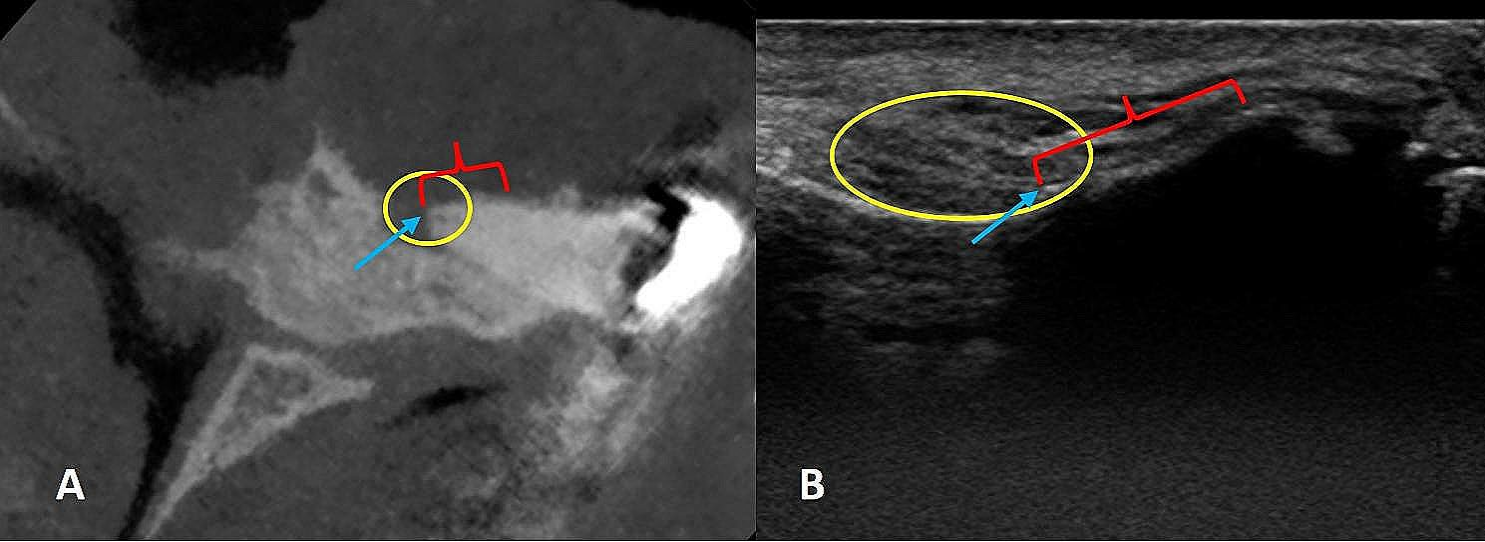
(A) CBCT image of a patient included in the study (which was taken for a different reason) shows a periapical lesion related with apex of right santral incisor teeth on cross-sectional view. (B) US image of the same tooth. The blue arrows indicate the root apex, the yellow circles denote the periapical region, and the red indicators define the root of the tooth. The CBCT image reveals a periapical lesion and bone resorption. In the US image of the same area, due to bone resorption, the ultrasonic wave has been transmitted the root and reflected back. Hence, the root apex (blue arrows) can be observed as hyperechoic within the apical lesion (yellow circle). Also, due to bone resorption in the relevant area, the ultrasonic wave has been able to reach the apical third of the root and reflected back. Therefore, the line indicated by the red marker appears more hyperechoic, while behind the opaque line, where the ultrasonic wave has not been transmitted beyond, no signal could be obtained, resulting in a anechoic appearance
